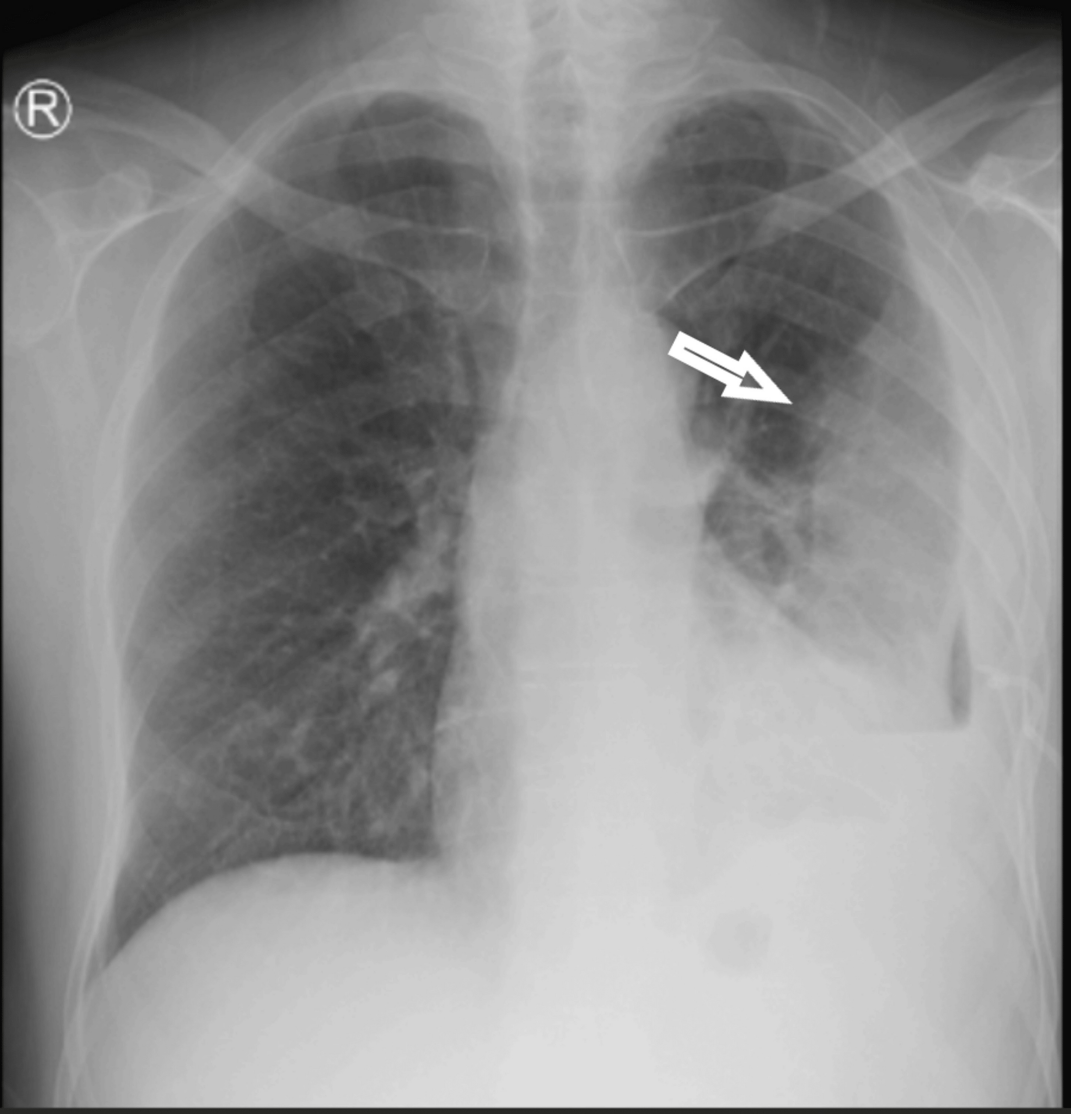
Chest X-ray. Chest X-ray demonstrates marked improvement in the left pleural effusion following pleural tapping.
